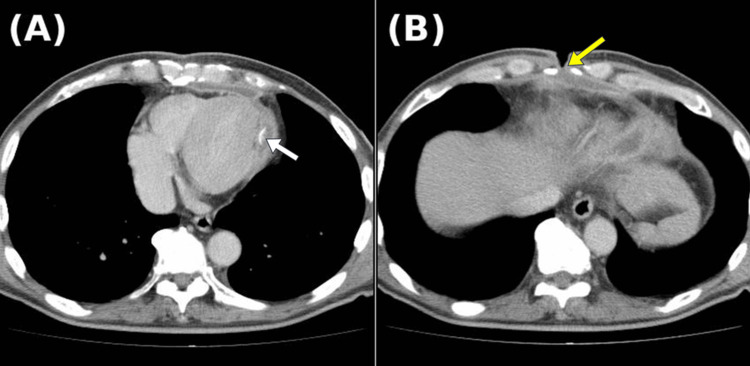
Preoperative computed tomography (CT) images (A) Residual Teflon felt is observed on the lateral wall of the left ventricle (white arrow), with encapsulated fluid extending along the anterior chest wall. (B) Communication between the subcutaneous incision site and the encapsulated fluid collection is visualized (yellow arrow).
